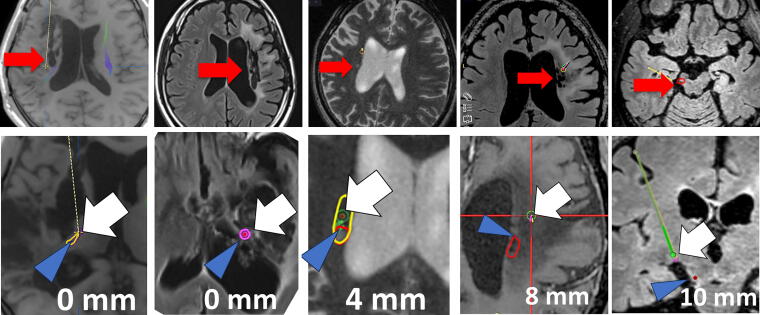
Representative figures of the patients with deep white matter damage. (Upper row) MRI axial image of the damaged area, where the red arrow indicates the actual damaged site. (Lower row) Probe eye views of the closest point between cell transplantation and the damaged area. The white arrow indicates the transplanted site, and the blue arrow indicates the closest point of the damaged area. The yellow area indicates the motor cortex, and the red circle indicates the responsible damaged area. MRI, magnetic resonance imaging.
